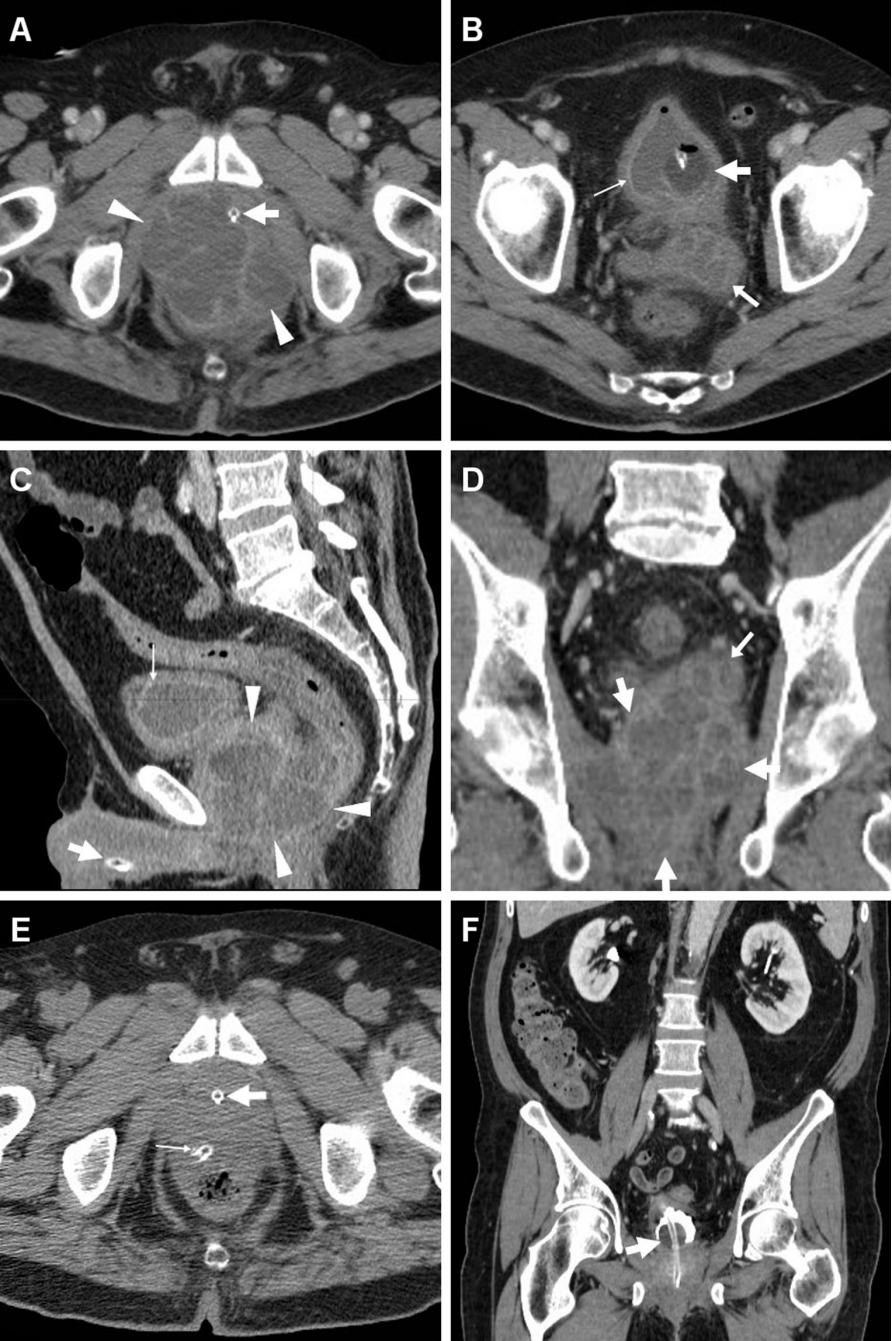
Large prostatic abscess from ESBL-positive *Escherichia coli* infection in a 61-year-old man with previous chemo- and radiotherapy for non-Hodgkin lymphoma, fever (38 °C), dysuria, pelvic pain and enlarged tender prostate at digital rectal examination. Multiplanar CT images (a–d) showed marked prostatic enlargement by confluent nonenhancing hypoattenuating (17–19 HU) regions, with peripheral and septal enhancement (*arrowheads*). The prostatic infection also involved the left seminal vesicle (*arrows* in b, d), displaced upwards of the urinary bladder, with mild circumferential mural thickening and mucosal hyperenhancement (*thin arrows*) consistent with UTI. After transperineal evacuation (e), follow-up CT urography (f) confirmed persistent resolution of the abscess [Partially reproduced from Open Access Ref. [47]]
